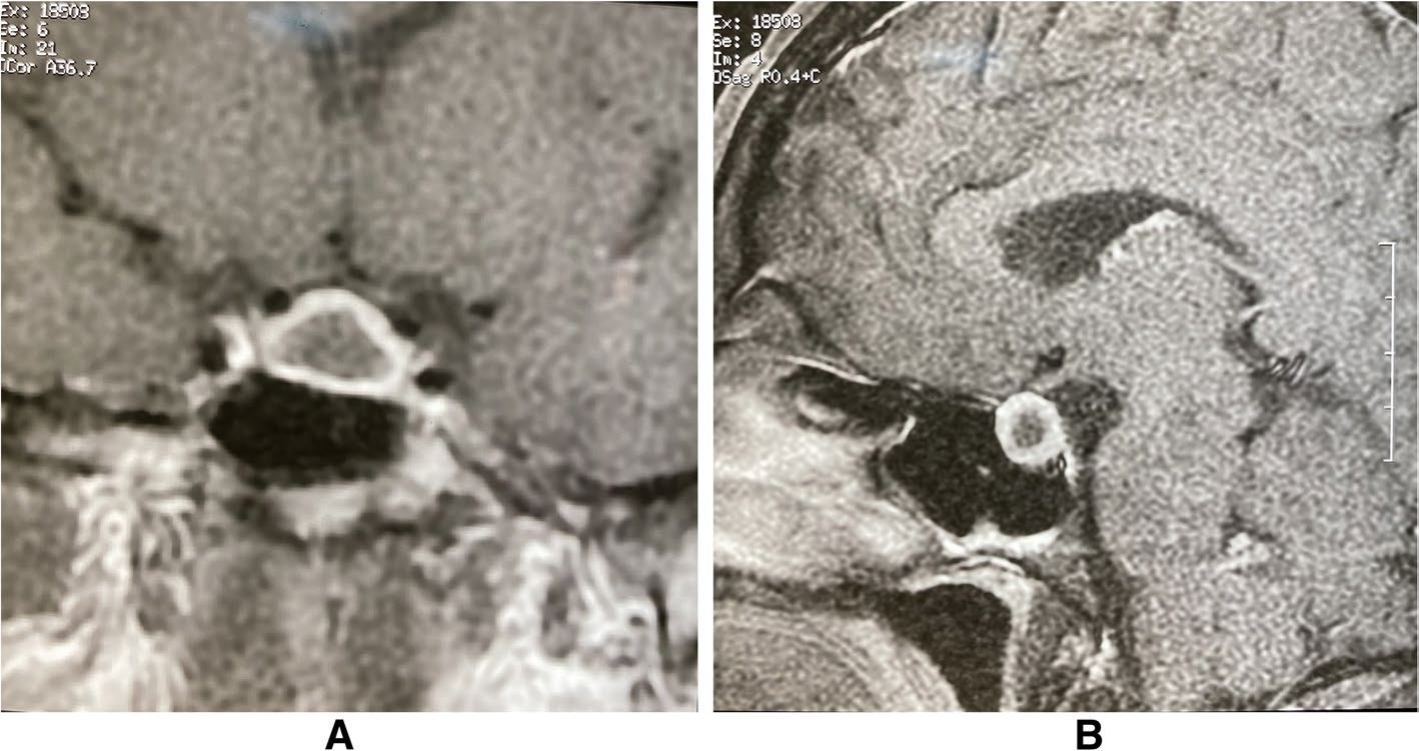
A, B Coronal (A) and Sagittal (B) post contrast T1-weighted images of pituitary gland demonstrate pituitary enlargement with rim enhancing hypointense lesion, superior pituitary convexity, and stalk thickening are also depicted
